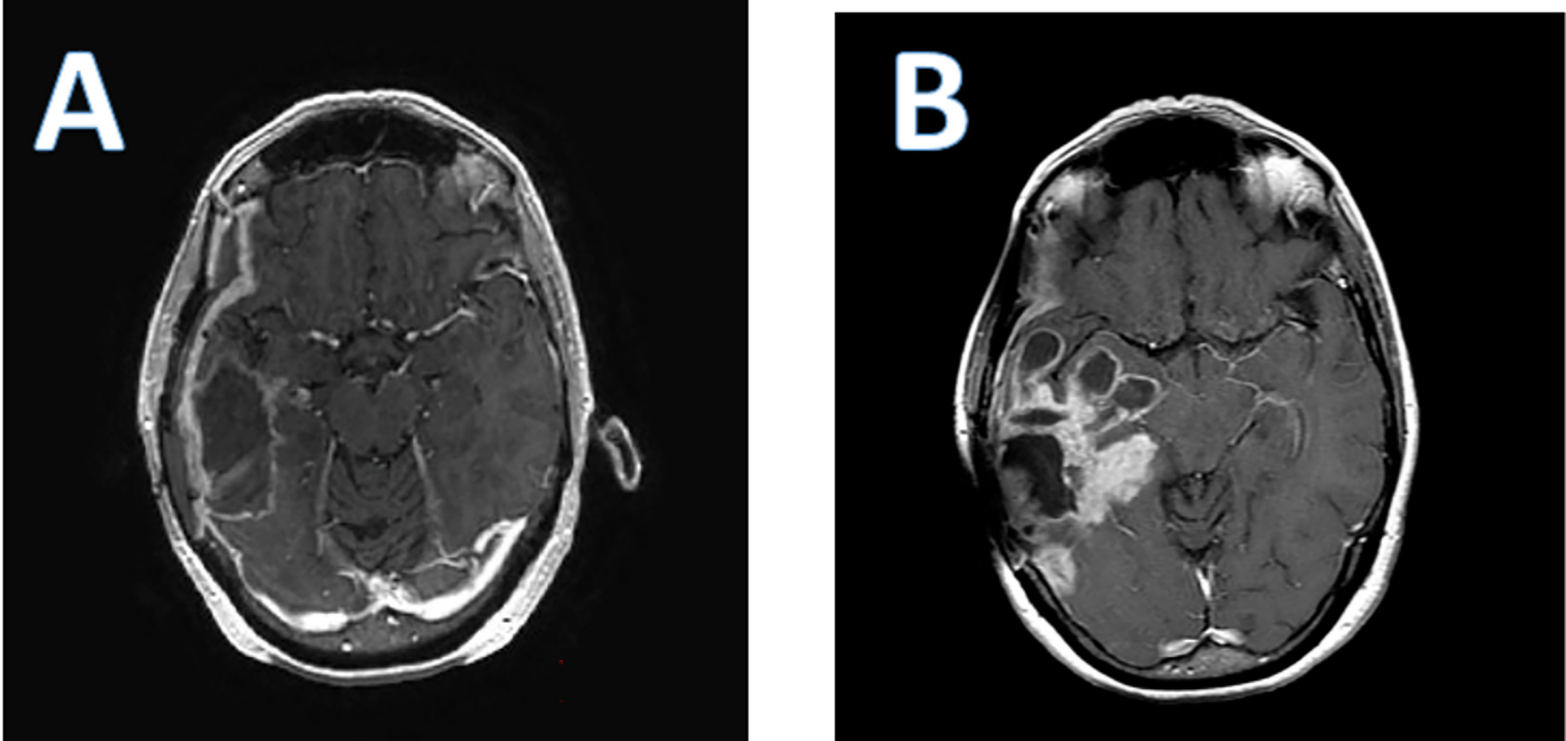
Axial T 1 post contrast enhanced brain MRI of a patient who received PVS-RIPO therapy. (A); baseline; (B); 4 months after PVS-RIPO, the MRI reveals cystic tissue degradation and parenchymal brain inflammation.
